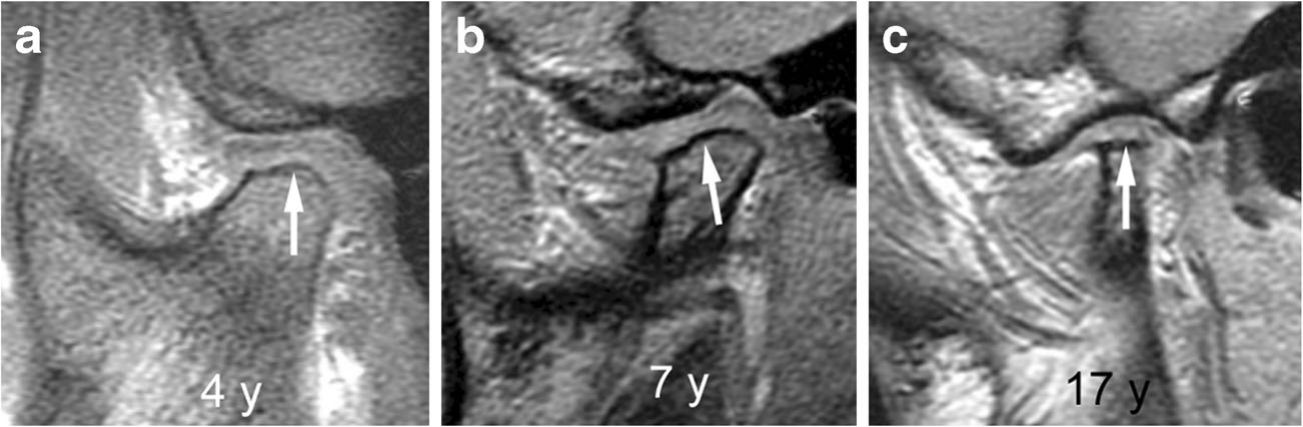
Severe condylar flattening (grade 2). a-c Sagittal oblique T1-weighted gradient echo images (TR/TE 300/4.2 ms, flip angle 80°, without fat saturation) from different children obtained at 4 years (a), 7 years (b) and 17 years (c) show flattening involving the entire surface of the condyle and horizontally oriented joint surface (arrows)
